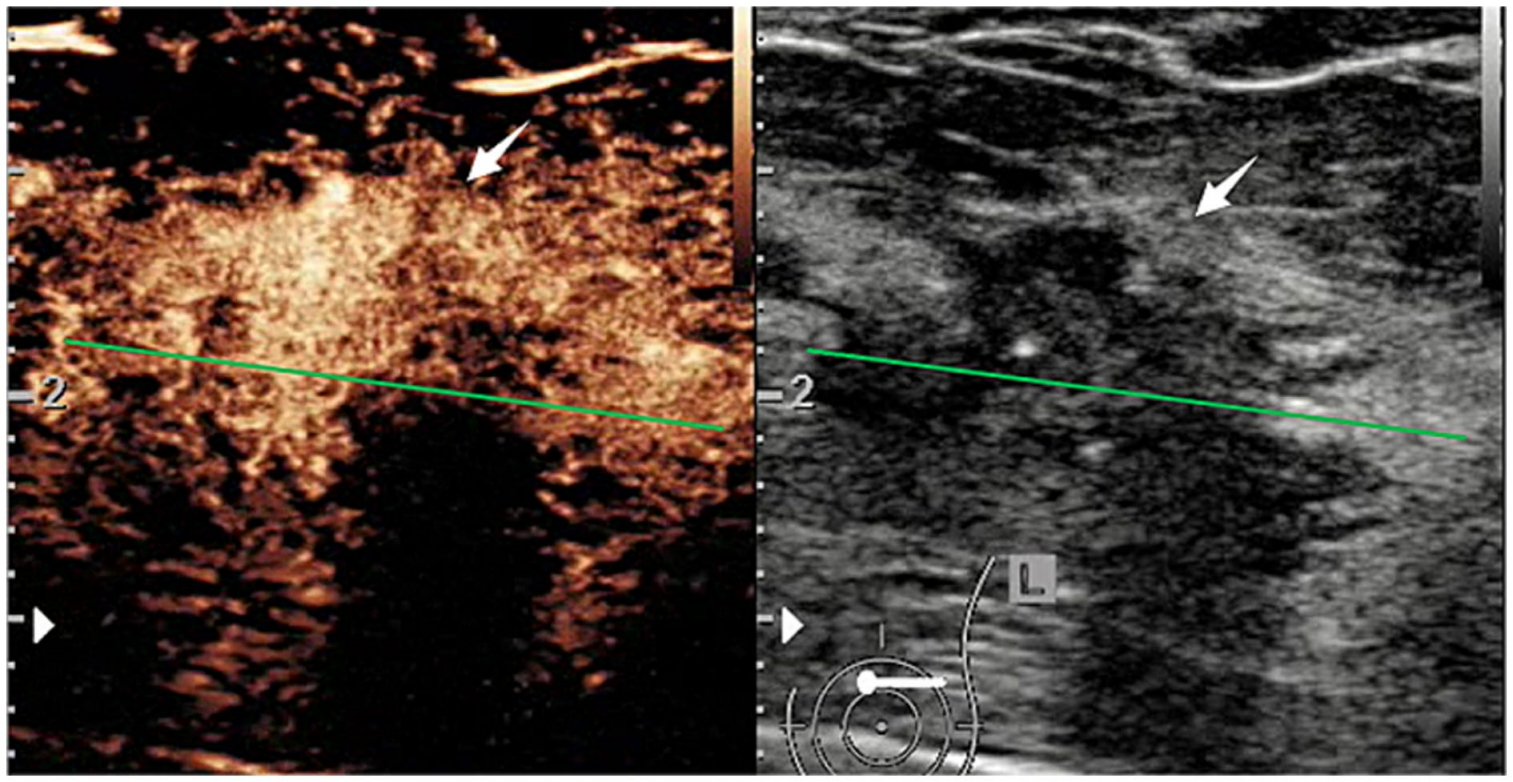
CEUS and B-mode ultrasound images of a patient with HER2-positive breast cancer.
